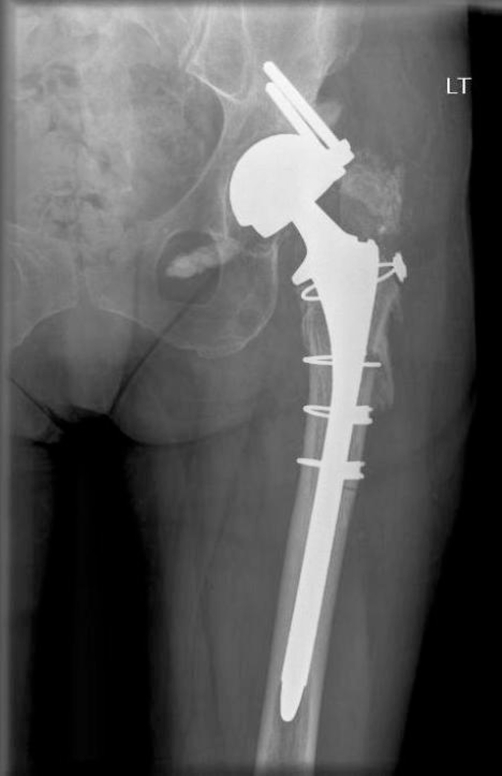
Initial postoperative radiograph showing a minimal interference gap between the acetabular cup and bone. Cup and screw positions are satisfactory.
